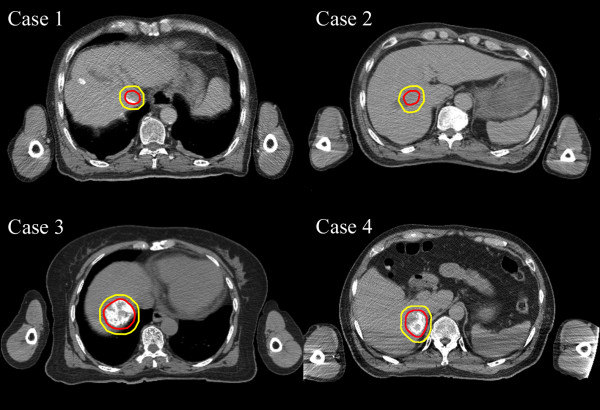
Planning computed tomography images. Outer and inner lines indicate the planning target volume and gross tumor volume, respectively. Case 1, hepatocellular carcinoma (23 mm) located in segment 1 (S1) near the duodenum; case 2, hepatocellular carcinoma (25 mm) located just below the diaphragm in S4; case 3, hepatocellular carcinoma (22 mm) located in S5 near the inferior vena cava and the duodenum; and case 4, hepatocellular carcinoma (40 mm) located in S6/7.
